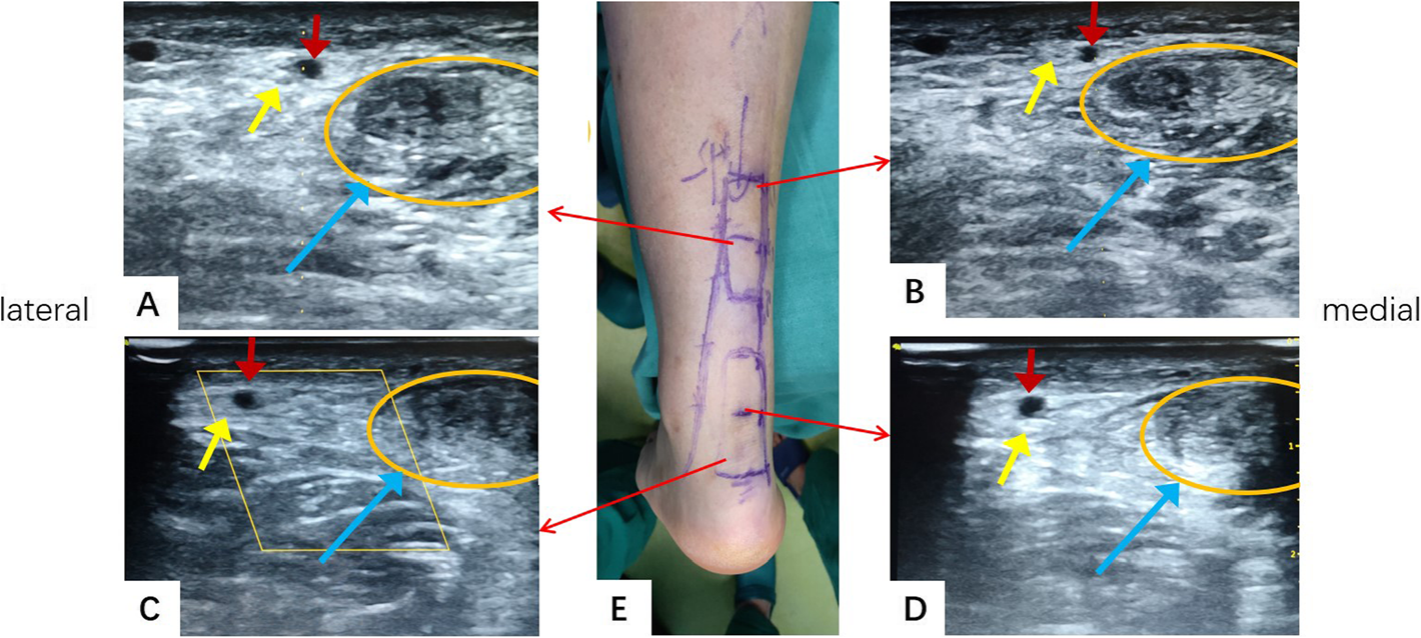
The intraoperative ultrasonography shows the relationship of the tendon, the SN, and the SSV at different levels from the insertion of the tendon on the calcaneus. It demonstrates that the SSV passes along the medial side of the SN. The distance between tendon and the SSV is shorter from the insertion of the tendon to the proximal side. The blue arrow shows the ruptured tendon; the red arrow shows the small saphenous vein; the yellow arrow shows the sural nerve
